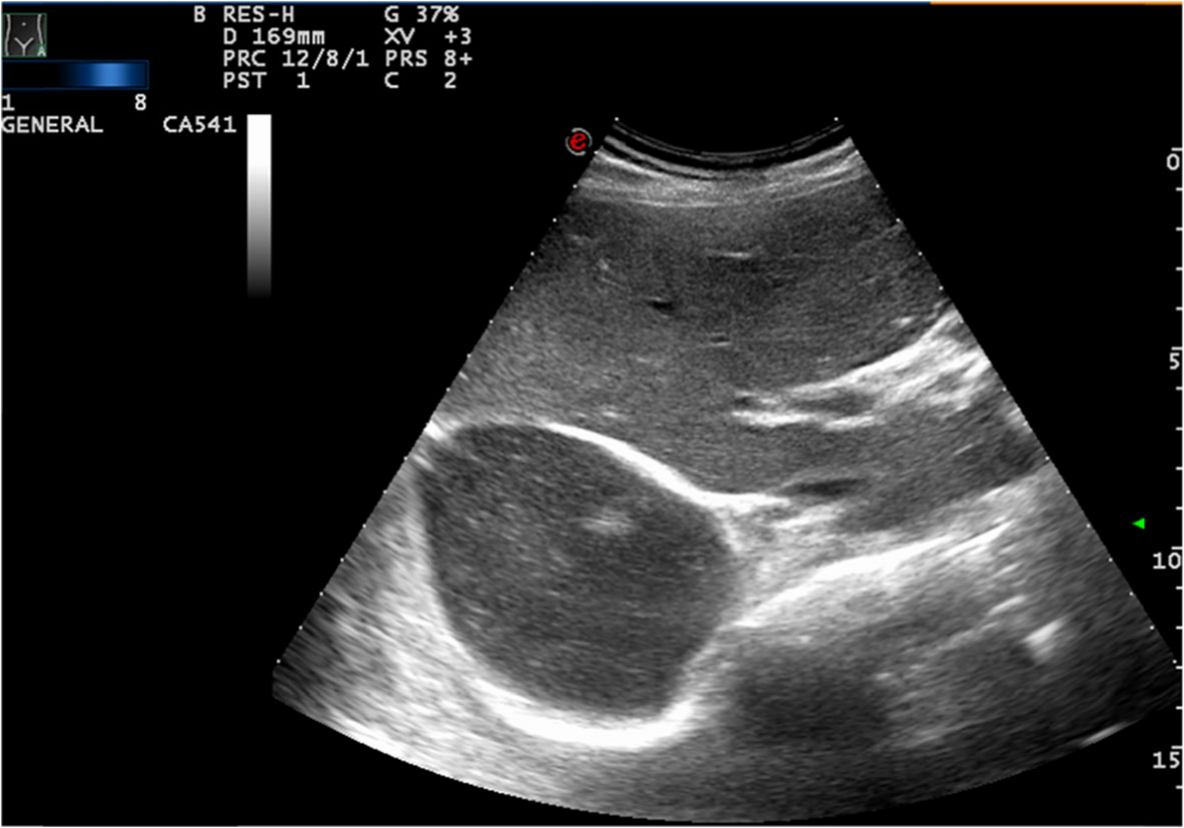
Ultrasonographic image of ALA in a male patient at Teaching Hospital Jaffna, demonstrating a solitary large right lobe liver abscess
